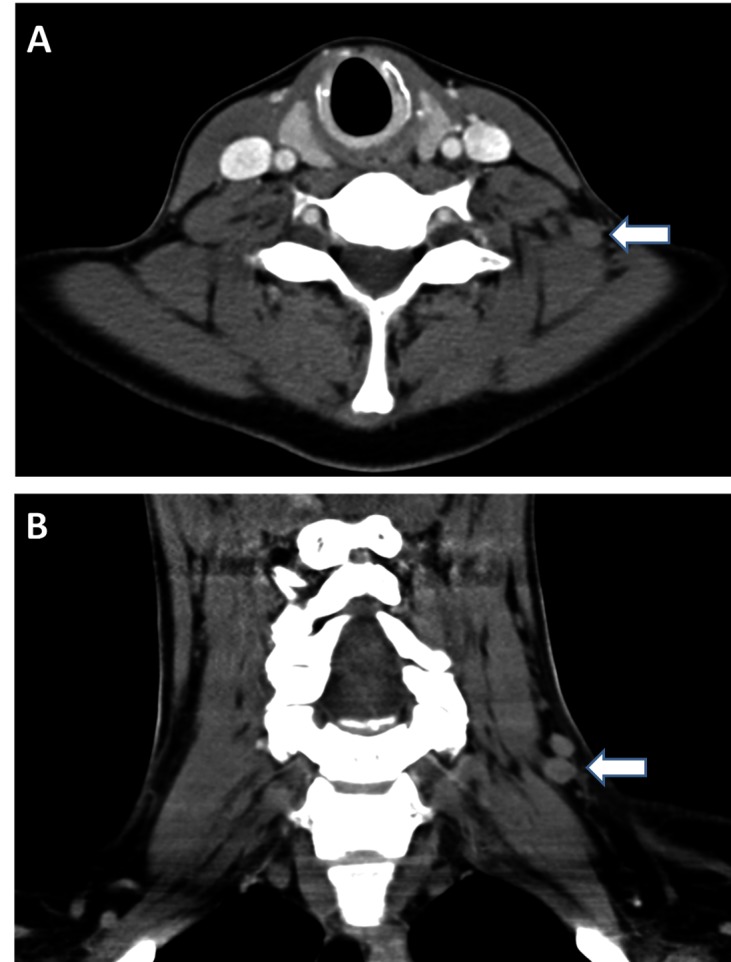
A soft tissue CT scan of the neck indicated lymphadenopathy A) Axial image at the level of the lower neck demonstrating a mildly enlarged left level 5B lymph node measuring up to 0.8 cm in the short axis. B) Coronal image at the level of the lower neck demonstrating two mildly enlarged left level 5B lymph nodes measuring up to 0.8 cm in the short axis.
